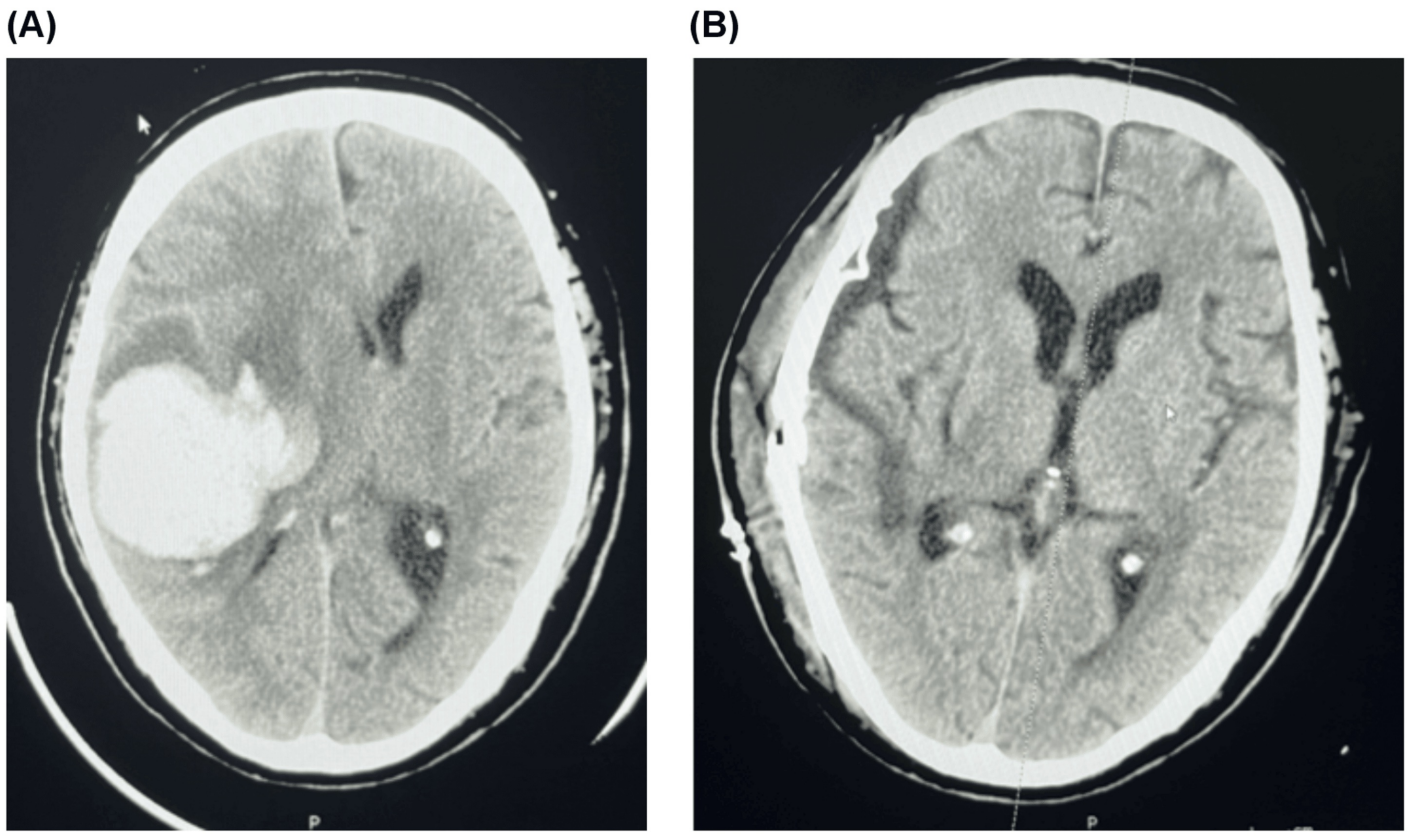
Axial CT images showing the patient’s right subcortical hemorrhage before and after surgical intervention (A) Axial image of preoperative CT scan, taken on day one, showing a right subcortical hemorrhage prior to craniotomy for hematoma evacuation. (B) Axial image of postoperative CT scan, taken after cranioplasty on day 40, showing the surgical results CT: computed tomography
